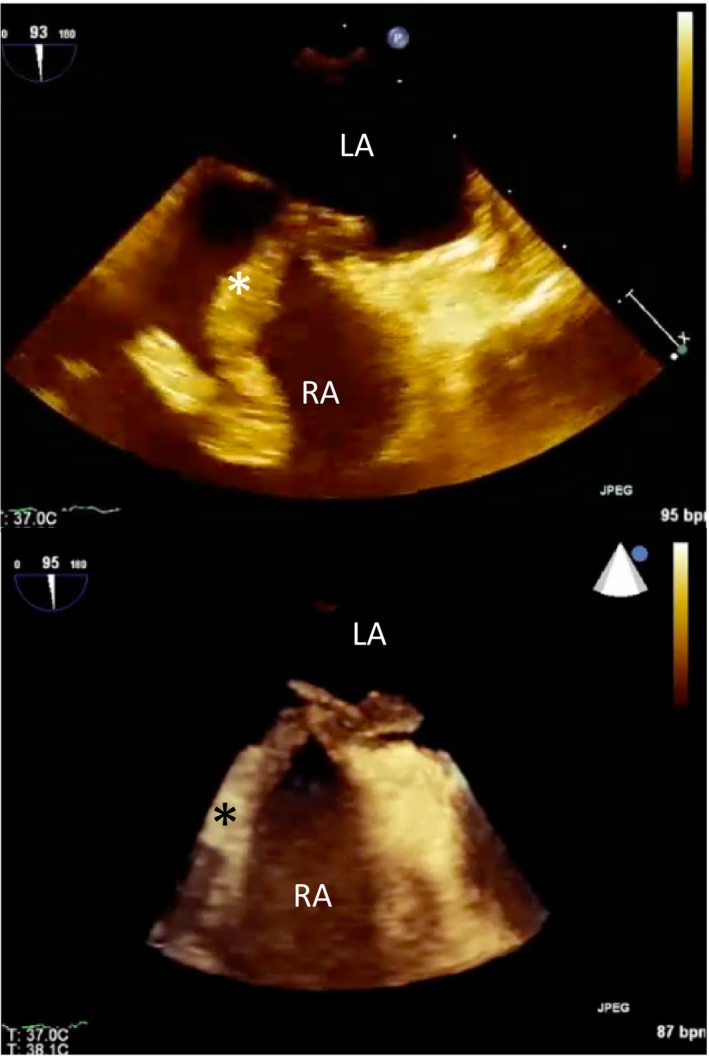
Clot in transit. Transesophageal echocardiogram images (2D and 3D) showing a large thrombus (*) in the right atrium (RA) traversing a patent foramen ovale (PFO) into the left atrium (LA). The patient suffered a fatal stroke.
